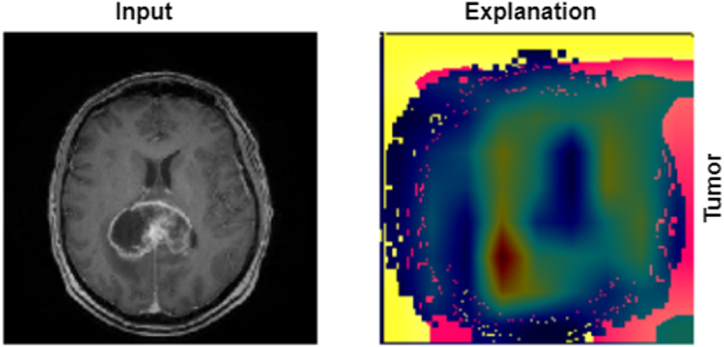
MRI brain scan (left) with corresponding Grad-CAM visualization (right) highlighting model focus areas for tumor detection.
